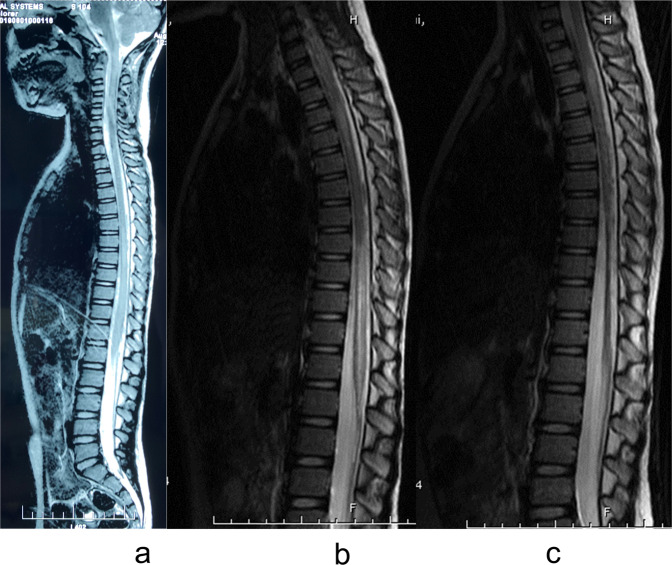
MRI of a 4-year-old girl with an injury to T4. a The day of injury with a slightly long T2 signal below T6. b One month later showing long signals at T3–4 and T8–L1 with a slightly long signal at T4–8. c Two months after injury showing a long signal at T3–4, a slightly long signal at T4–8, and spinal cord atrophy below T8.
